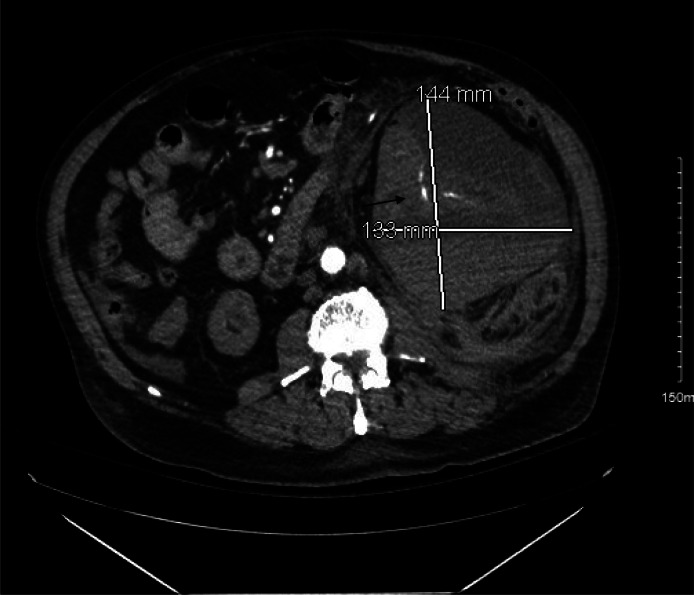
Axial view of computed tomography angiogram of the abdomen showing large left renal hematoma with contrast blush (arrow) suggestive of active bleeding. *mm*, millimeter.
